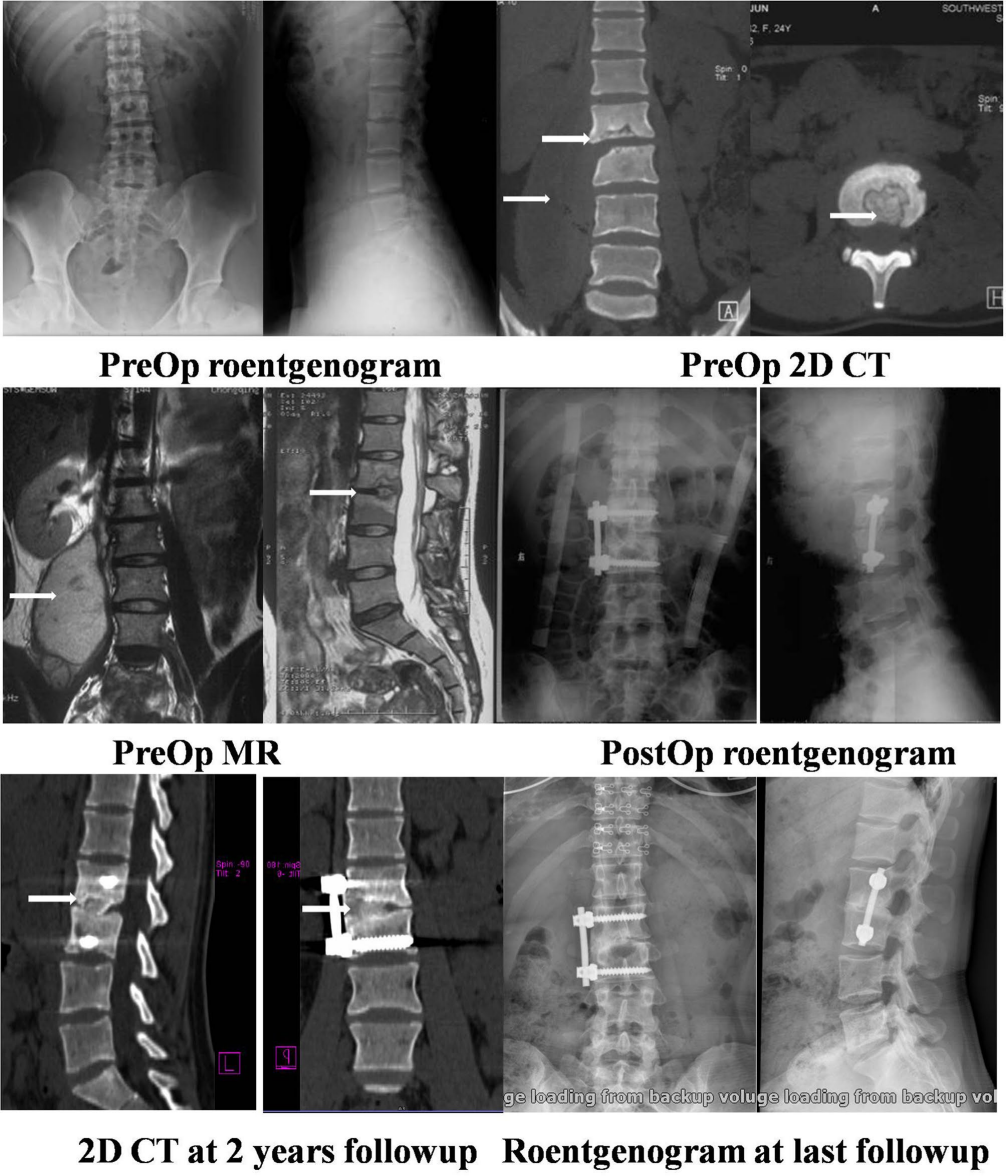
A 25-year-old woman complained of severe back pain. The preoperative roentgenogram, 2D CT scan and MRI scan showed destruction of L2/3 vertebral bodies and right psoas abscess (white arrows). The immediate postoperative roentgenogram showed the tricortical iliac crest allograft and anterolateral single rod instrumentation. The 2D CT scan taken at the 2-year follow-up showed good incorporation and normal lordosis. The roentgenogram taken at the 9-year follow-up showed that solid bony fusion was achieved, and the loss of correction was 4°.
